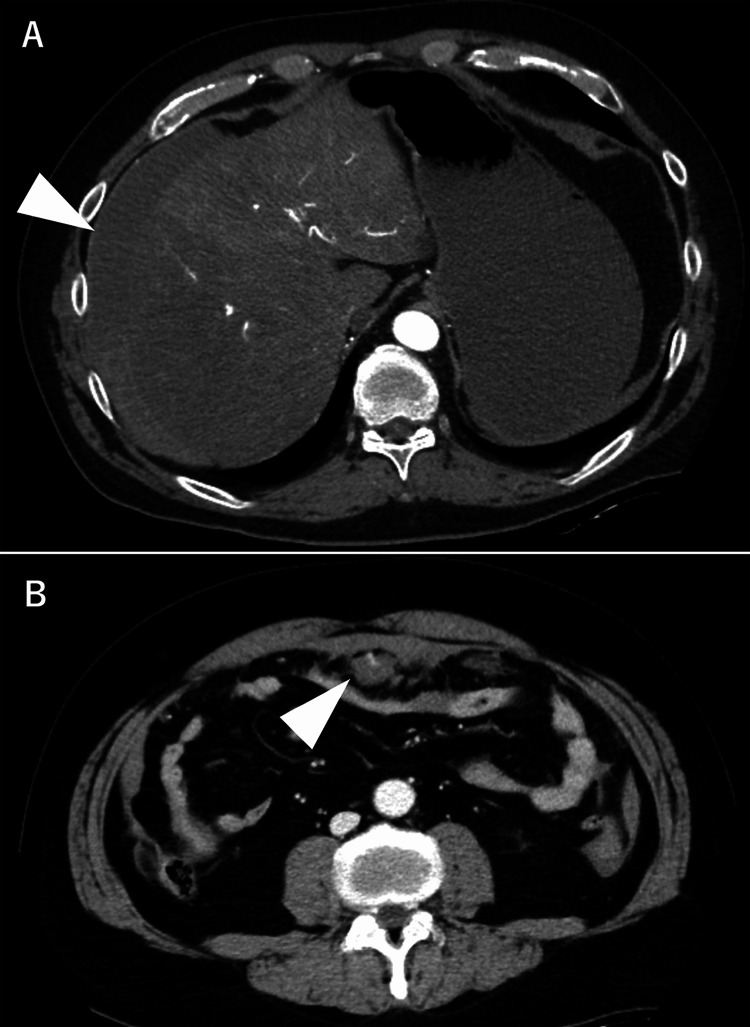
Contrast-enhanced CT findings on day 1 A. Contrast-enhanced CT at admission showing hemoperitoneum on the liver surface(arrow). B. Contrast-enhanced CT revealing a hematoma in the fat tissue with contrast extravasation inside (arrow) CT: computed tomography
